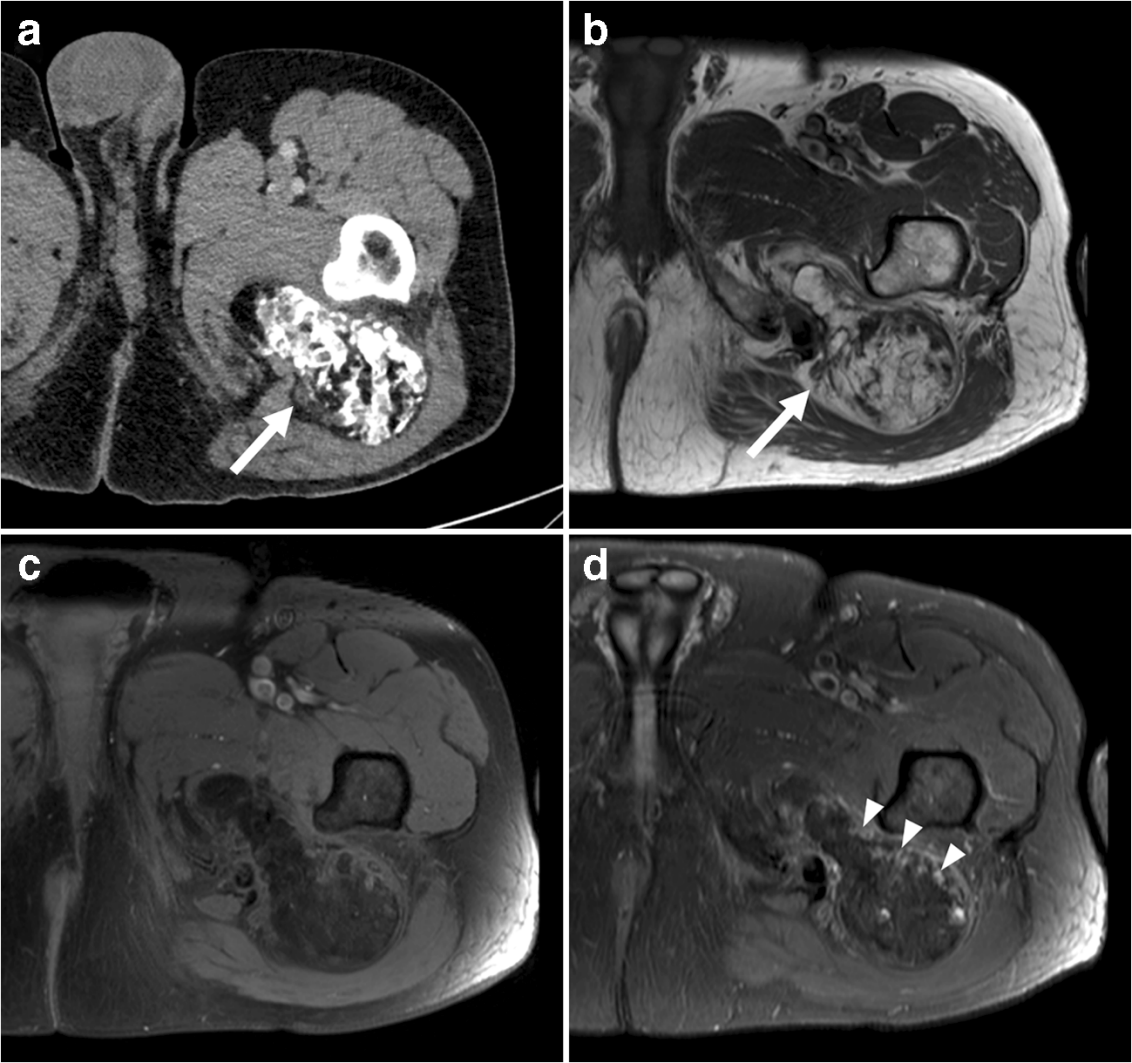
A 68-year-old man with lipoma with metaplastic bone. a Axial CT shows a well-defined mass (*arrow*) with ossifications intermixed with fatty tissue in the left ischiofemoral space. b Axial T1-weighted image shows the fatty tissue within the mass. c, d  Axial pre- (c) and postgadolinium (d) T1-weighted images with fat suppression show enhancing areas in the anterior part of the mass (*arrowheads*). Pathological analysis after resection confirmed lipoma with metaplastic bone
